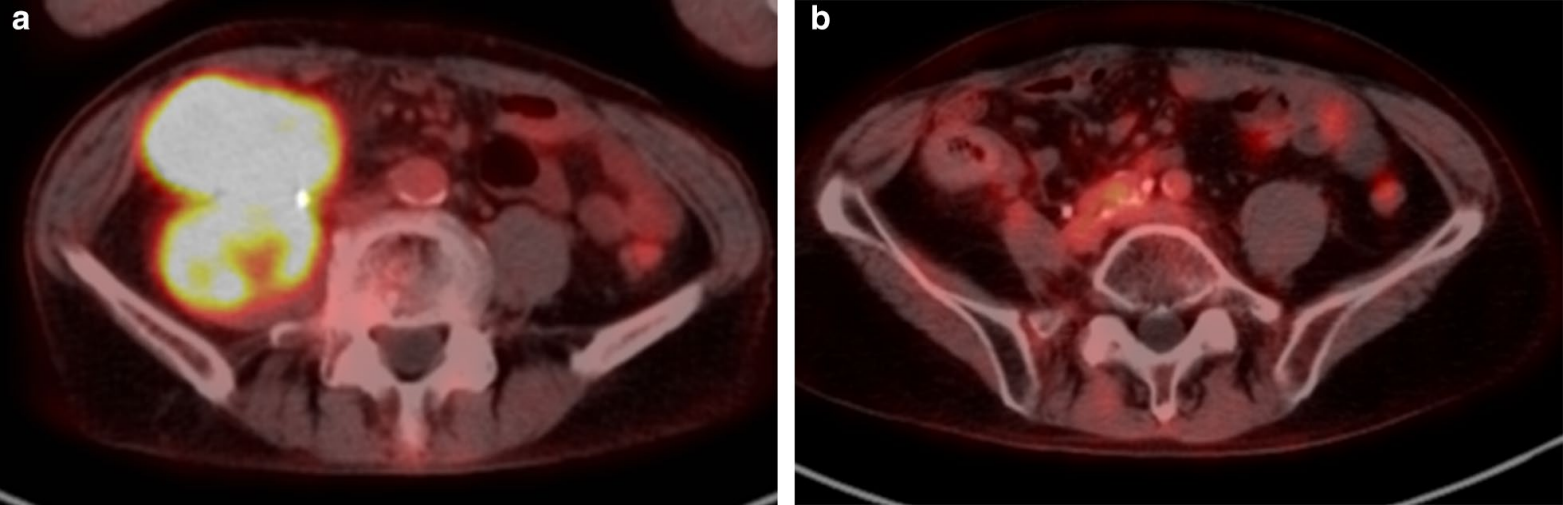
PET–CT showed that there was strong uptake to main tumor and surrounding lymph nodes (a). After 20 courses of immunotherapy, there was no abnormal uptake in all tumors, which was diagnosed as cCR (b)
